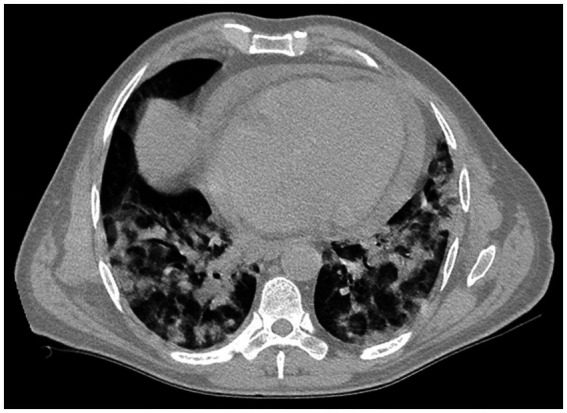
High-resolution computed tomography of the chest showing moderate pericardial effusion obliterating the anterior, posterior, and lateral recesses. The parenchyma of both lungs shows multi-lobar ground-glass opacities with no preferential predominance, superimposed with sub-pleural bands of atelectasis, interlobular septal thickening, and crazy-paving pattern typical of COVID-19 pneumonia.
